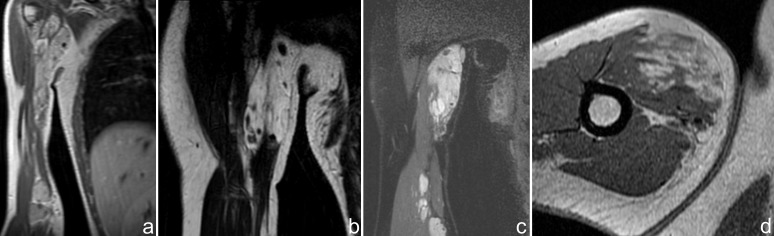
MRI exam showing two large soft tissue masses, localized in the upper and lower brachial muscles, with the same signal intensities on T1 and T2 sequences (a,b) and extension in the anteromedial axillary zone (b). Similar areas with high signal on STIR sequences were observed between these two tumors (c). The tumors enhance heterogeneous with signal voids corresponding to calcifications (d)
